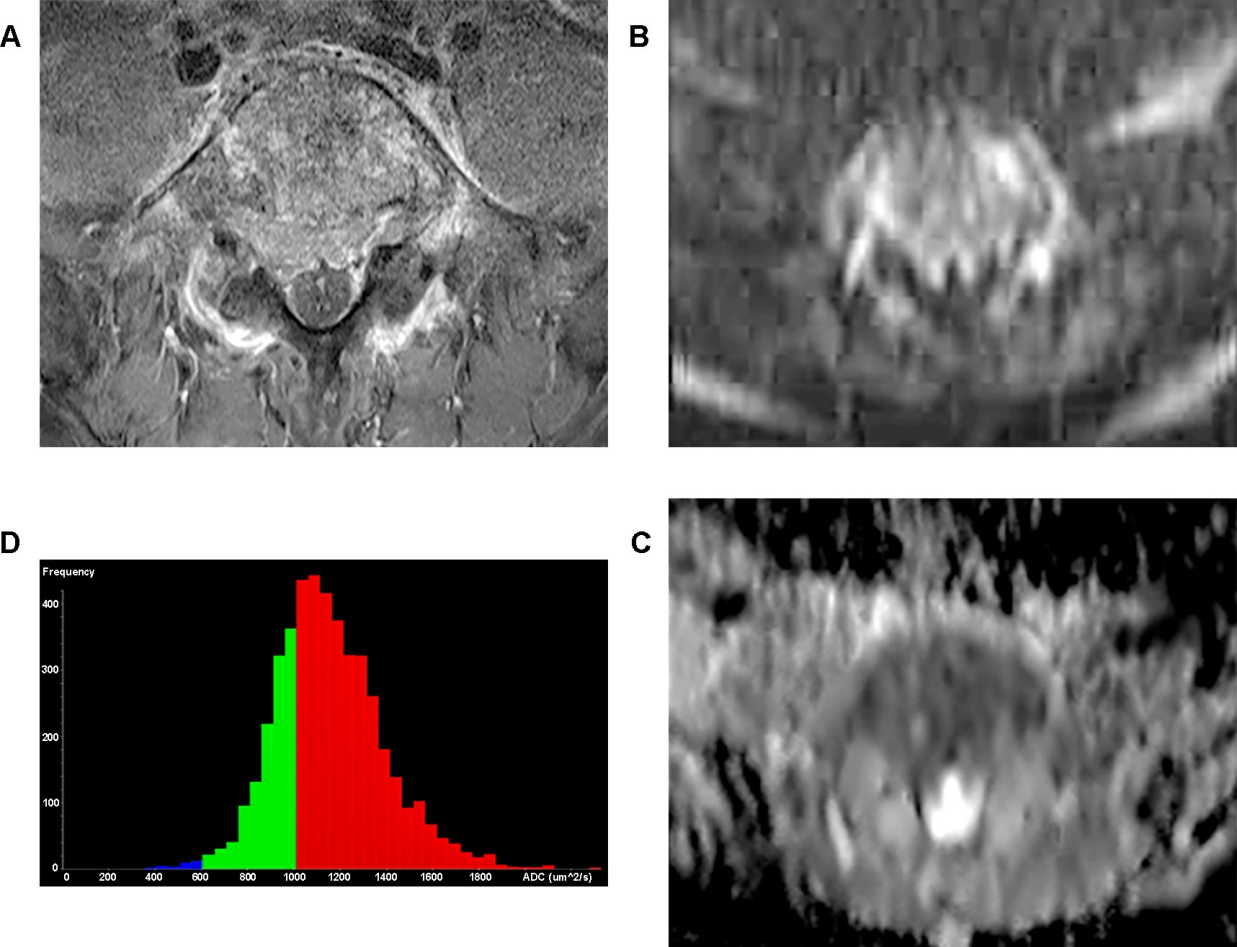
Representative case of metastasis. A 61-year-old man with metastasis from hepatocellular carcinoma. (a) Axial fat suppressed contrast-enhanced T1-weighted image shows pathologic fracture of L5 vertebral body. (b) Axial DW image (b = 800 sec/mm^2^) at the same level reveals hyperintense signals. (c) Corresponding axial ADC map (b = 0 and 800 sec/mm^2^) shows low signal intensity. Median value of ADCav was 1110 ± 150 μm^2^/sec, ADCmin was 1003 ± 57 μm^2^/sec. (d) In the corresponding ADC histogram of whole tumor volume, mean value of ADCvol was 1155 μm^2^/sec and SDvol was 236. Interpretations were correct for both readers.
